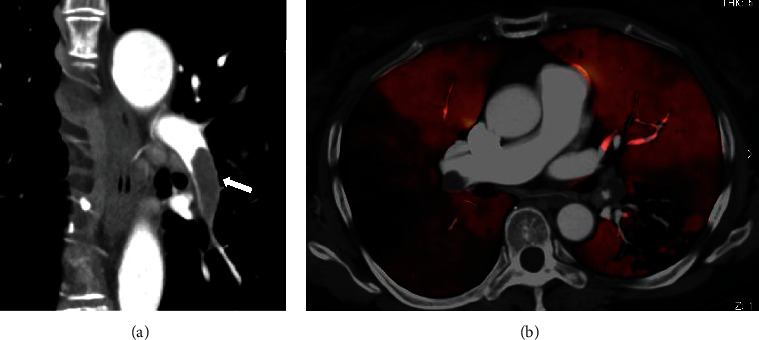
(a) Contrast-enhanced computed tomography (CT) reveals a large lesion filling the left pulmonary artery (white arrow). (b) Dual-energy CT shows areas of severe hypoperfusion in segment S4 of the right lung and segment S6 of the left lung.
